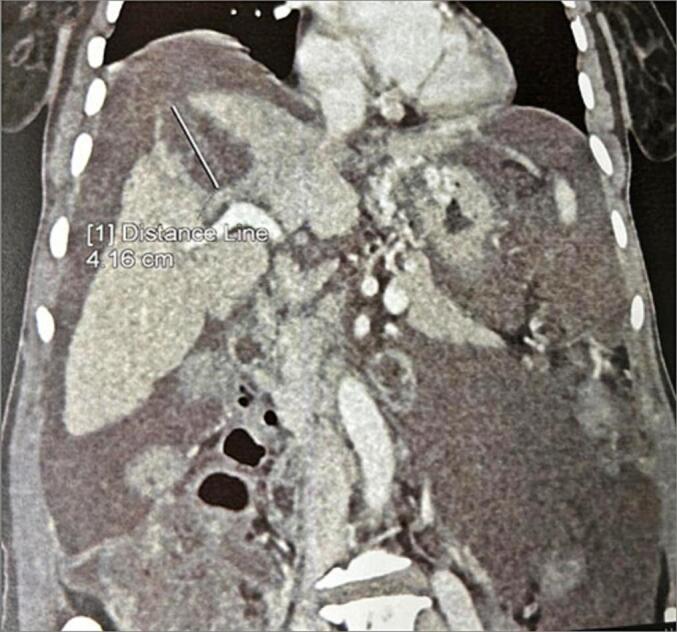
Abdominal CT scan showing a dysmorphic liver with a ruptured hydatid cyst in segment VIII (white arrow) and associated peritoneal effusion (asterisk).
